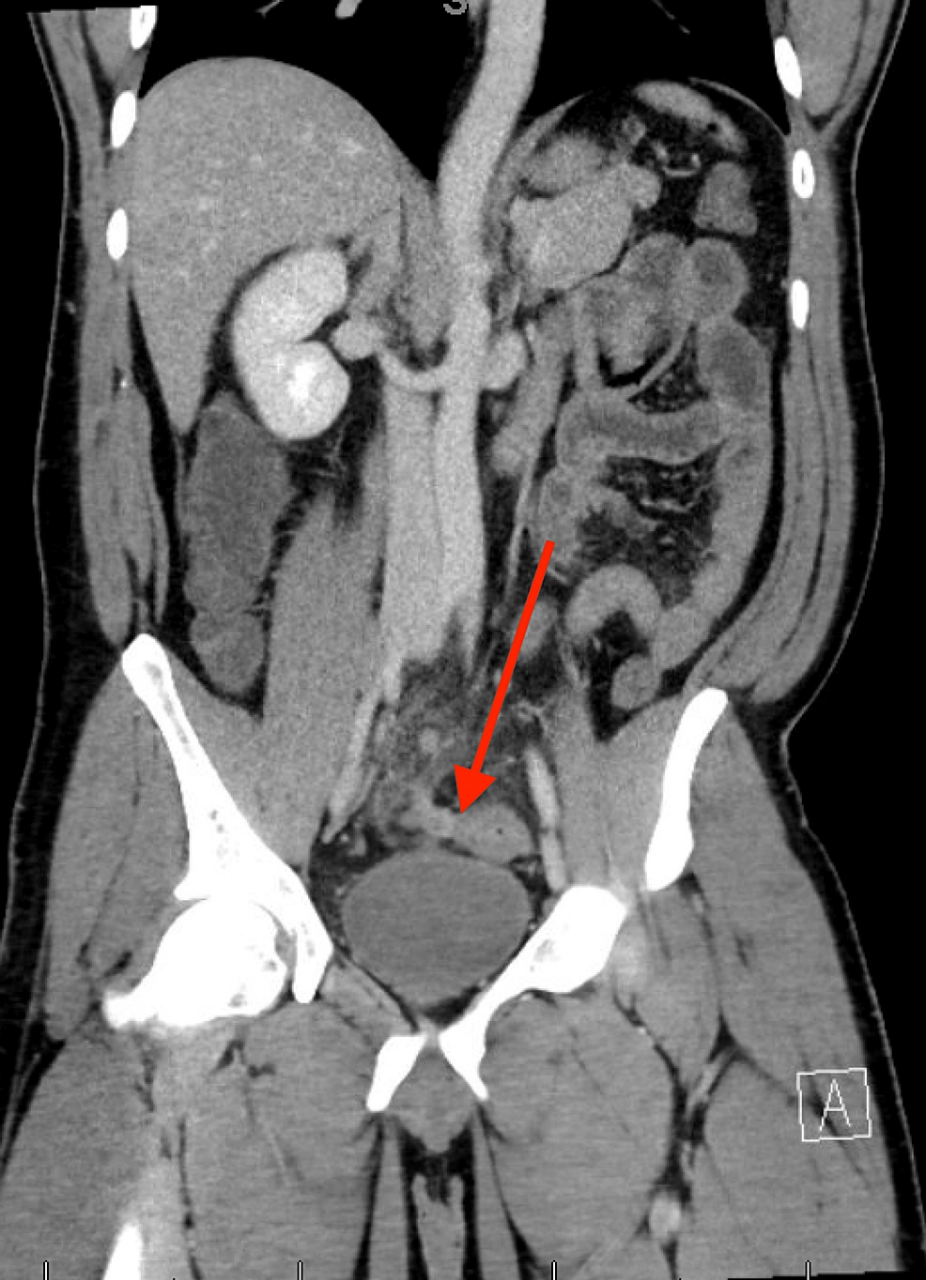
Coronal image of the CT on admission showing the close approximation of the appendix with the sigmoid colon.
